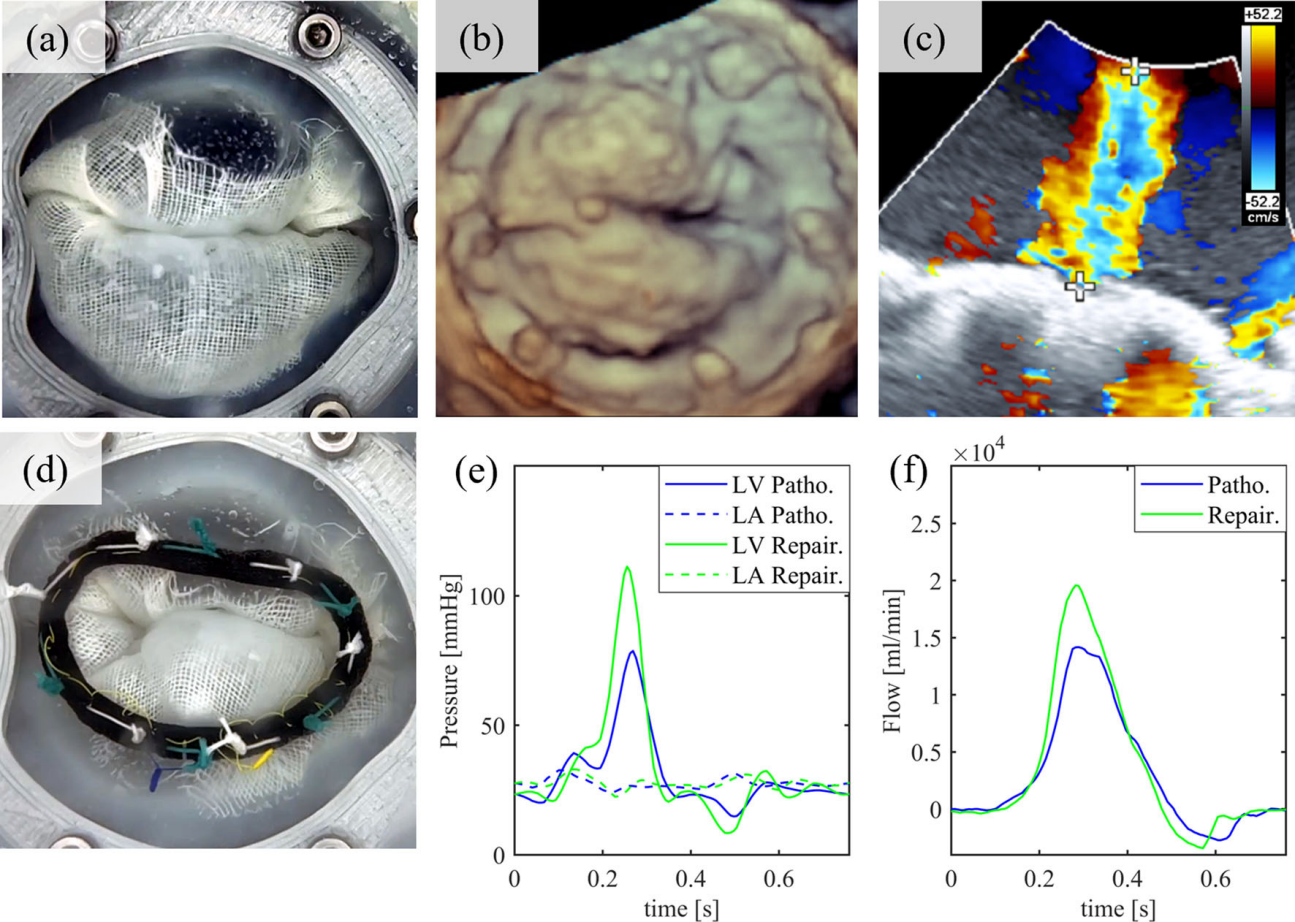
a–d In-vitro valve with bi-leaflet prolapse pathology (a), 3D-ultrasound image (b), regurgitation jet in color-Doppler mode (“+–+”) (c), and after MIMVS (d). e, f Pressure (e) and Flow (f) graphs. LV, left ventricle; Patho., pathological condition LA, left atrium; Repair., repaired condition
